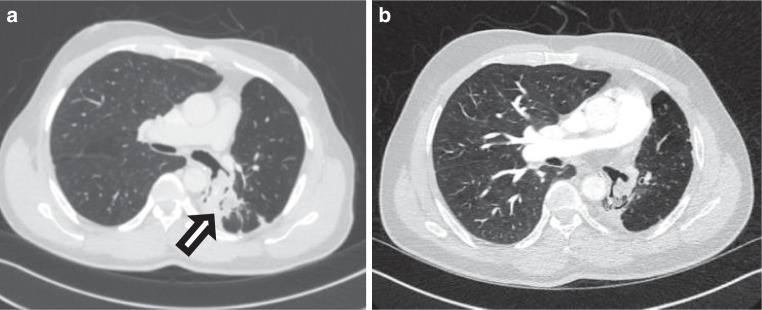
Pre- and postradiotherapy (RT) imaging. a Pre-RT thoracic CT scan 8 years after partial surgical resection of the left inferior lobe. The aspergilloma is localized adjacent to the great pulmonary vessels, showing the characteristic radiographic feature of the “air-crescent sign” (*white arrow*). b Aspergilloma decreased in size at 3‑year follow-up and no further interventions were necessary. After mild episodes of hemoptysis initially, no further episodes have been reported since then. *CT* computed tomography*, RT* radiotherapy
